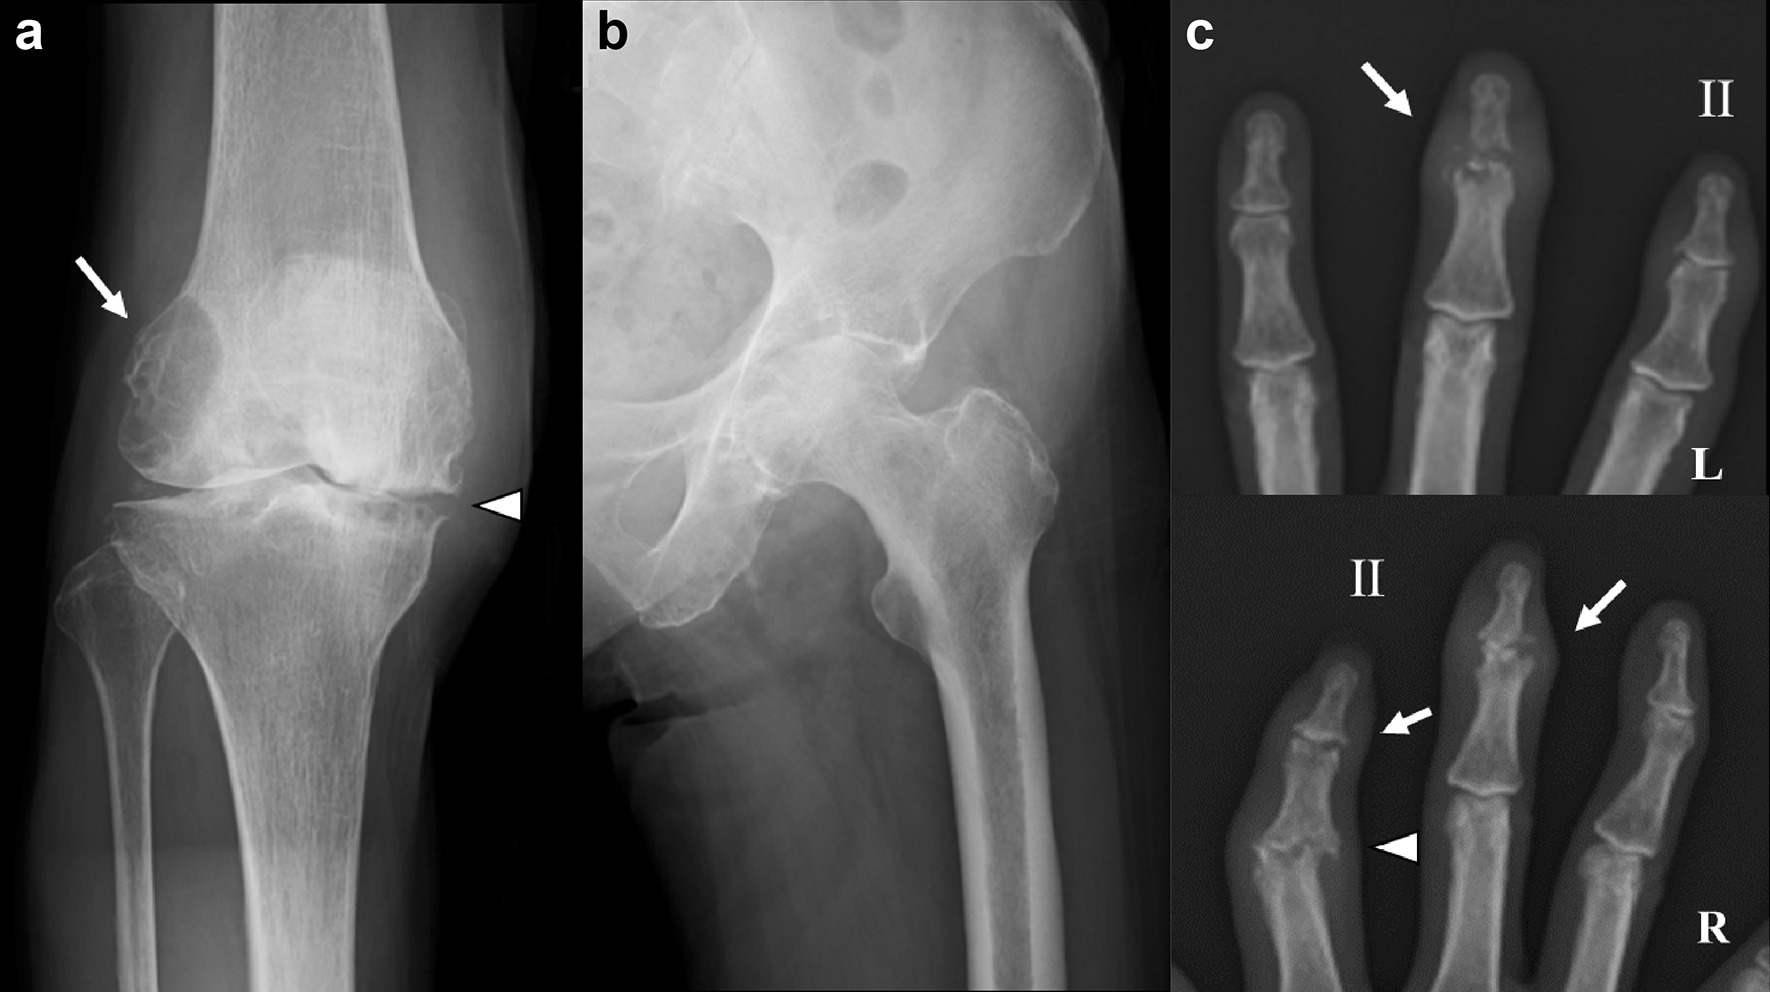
X-ray findings at the first visit. a Anteroposterior radiograph of the knee. Bone cyst formation in the lateral condyle of the femur (arrow) and joint destruction of the medial compartment (arrowhead). b Narrowing joint space of the left hip. c Pencil cup-like destruction of the distal interphalangeal joint (arrow) and destruction of the proximal interphalangeal joint (arrowhead)
